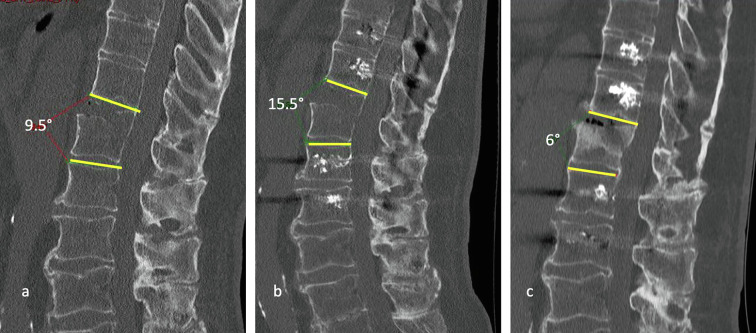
CT scan of a 75-year-old patient in the pre-operative (a), immediate post-operative (b), and 6 months post-operative (c) stages, who underwent cemented percutaneous long posterior instrumentation for a T11–T12 fracture. Despite the absence of an anterior procedure, there is a progressive correction of the anterior diastasis at 6 months: preoperative lordotic angulation was 9.5°, immediate postoperative angulation was 15.5° and angulation at the late postoperative stage was 6°.
